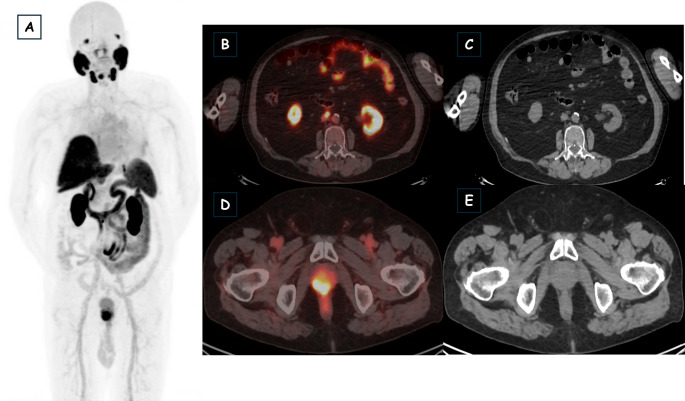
A 78-year-old man underwent ^18^F-PSMA-1007 PET/CT in our department due to biochemical recurrence. There were no suspicious lesions on abdominal CT despite rising PSA levels from 4.25 ng/mL to 5.57 ng/mL within a 3-month interval. The patient had been subjected to locoregional radiotherapy at the prostate bed and T9-T11 four years earlier and subsequently been treated with androgen deprivation therapy. The initial Gleason score was 8 (4 + 4). MIP (Panel A) and axial images (Panels B and D) show local relapse and a solitary extrapelvic (RP) lymph node metastasis, both with ^18^F-PSMA-1007 uptake higher than spleen but obscure on CT (Panels C and E). PROMISE code: miTr(3a)N0M1a, PSMA expression score lowest 2 (other lymph nodes not herein shown) and highest 3
